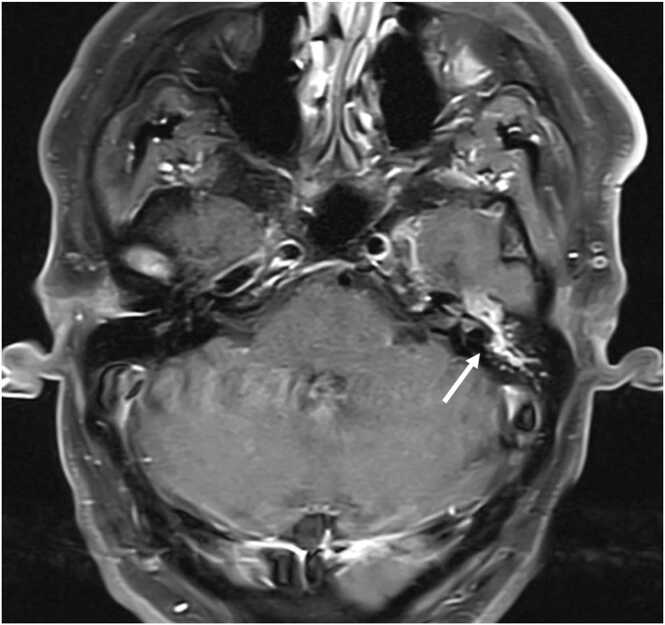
T1 fast spin echo brain magnetic resonance imaging showing soft tissue or fluid density material within the left middle ear cavity and left mastoid air cells as well as bony demineralization of the ossicular chain (as denoted by the white arrow).
